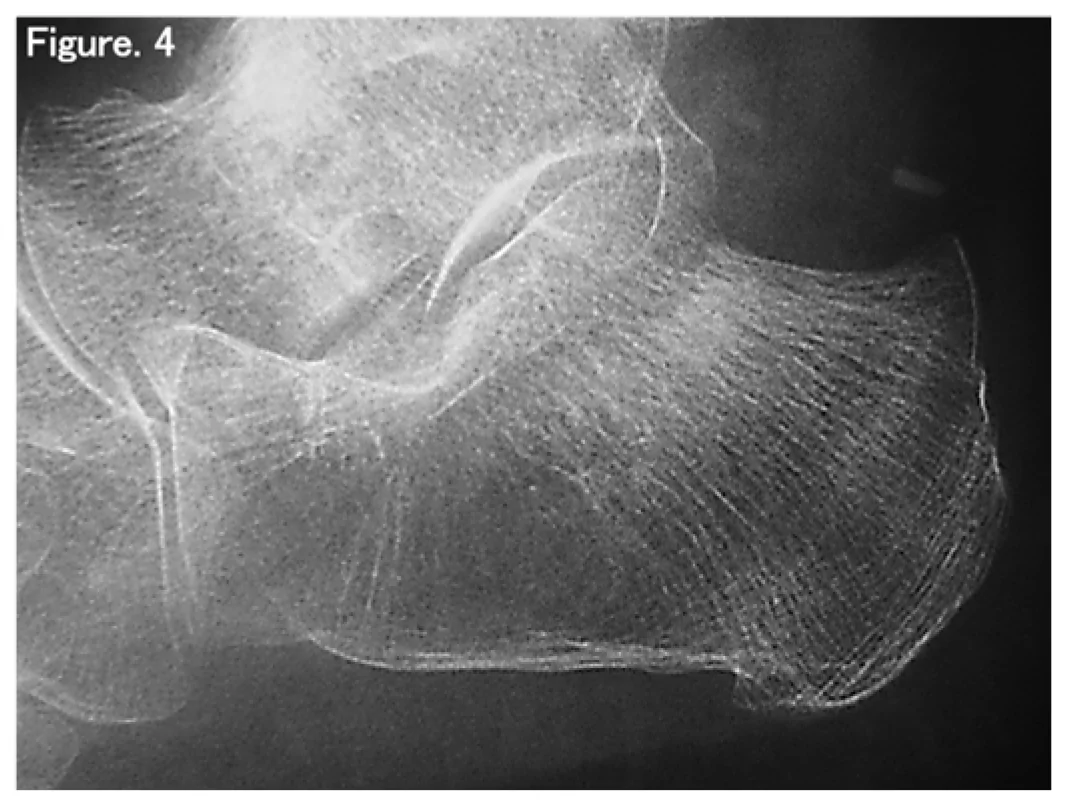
Follow-up radiograph of the same 74-year-old woman. Osteosclerotic change resolved during follow-up after conservative treatment.
